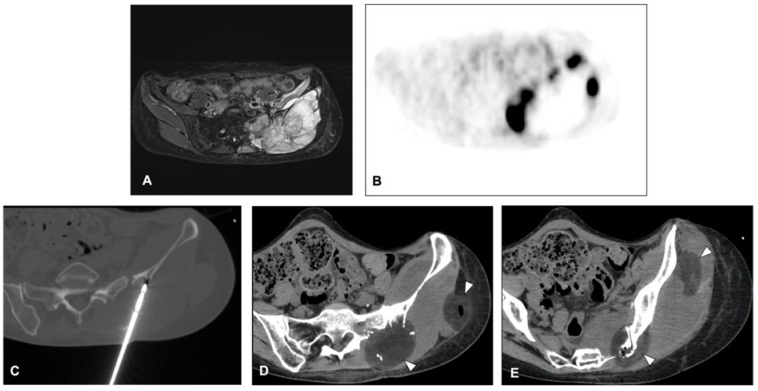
Axial T2 MR image of a 51-year-old woman with metastases from leiomyossarcoma (A) in the pelvis treated with cryoablation (C) for palliative intent. 18F-FDG PET/CT scan performed before the procedure demonstrates the pathologic radiotracer uptake in areas of viable tumor (B). The procedure was performed in an attempt to cover these areas and the post-procedure ice balls are visible as hypodense circles (arrowheads—(D,E)).
